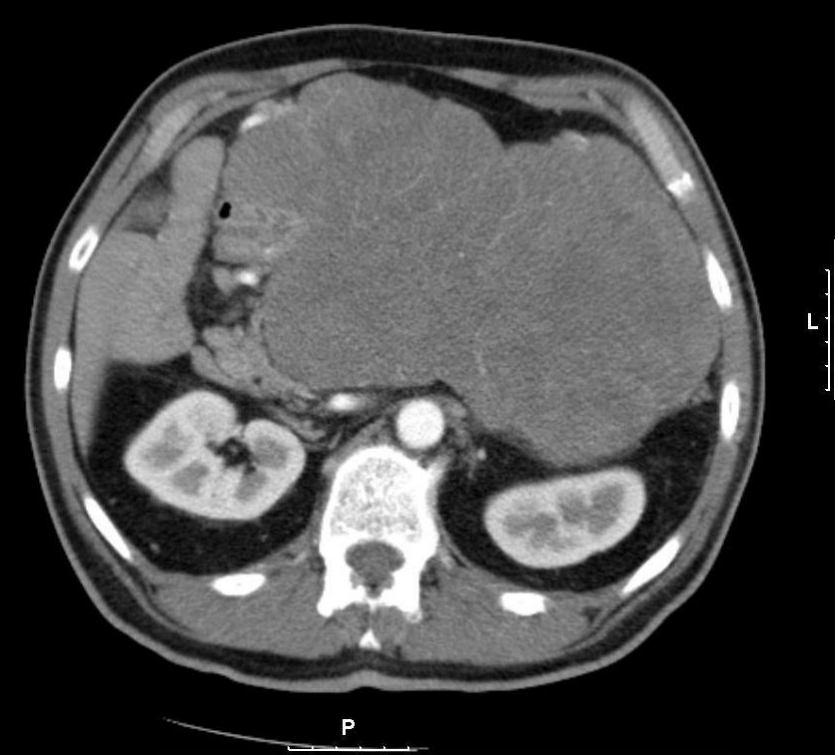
Initial CT scan revealing an abdominal mass measuring 21 × 12 cm.
